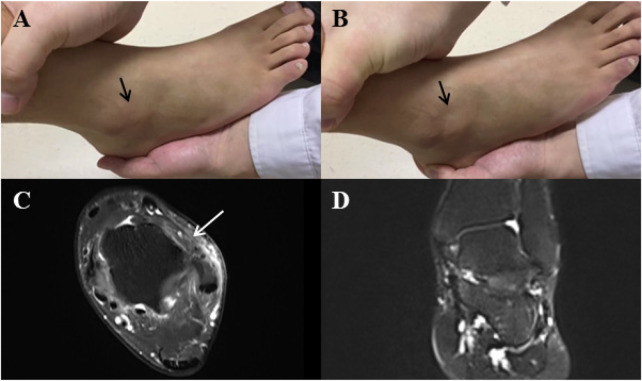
(A) before the anterior drawer test of the ankle, the area in front of the lateral malleolus near the anterior talofibular ligament is filled. (B) During the anterior drawer test, the talus moves forward, and the area where the anterior talofibular ligament runs becomes depressed (black arrow). (C) T2-weighted MRI of the ankle revealed a rupture in the anterior talofibular ligament (white arrow). (D) T2-weighted MRI of the ankle revealed no evidence of damage to the articular cartilage.
